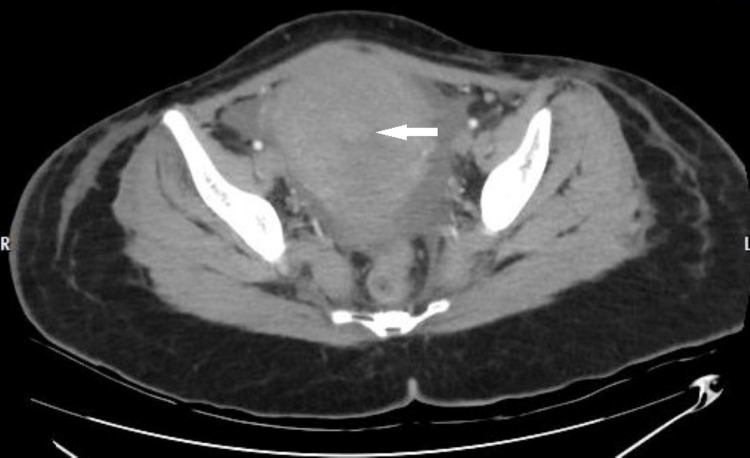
CECT pelvis showing a bulky uterus with heterogenous hyperdense content in the endometrial cavity, suggestive of retained products of conception, and no evidence of IUCD. CECT - contrast-enhanced computed tomography; IUCD - intra-uterine contraceptive device.
